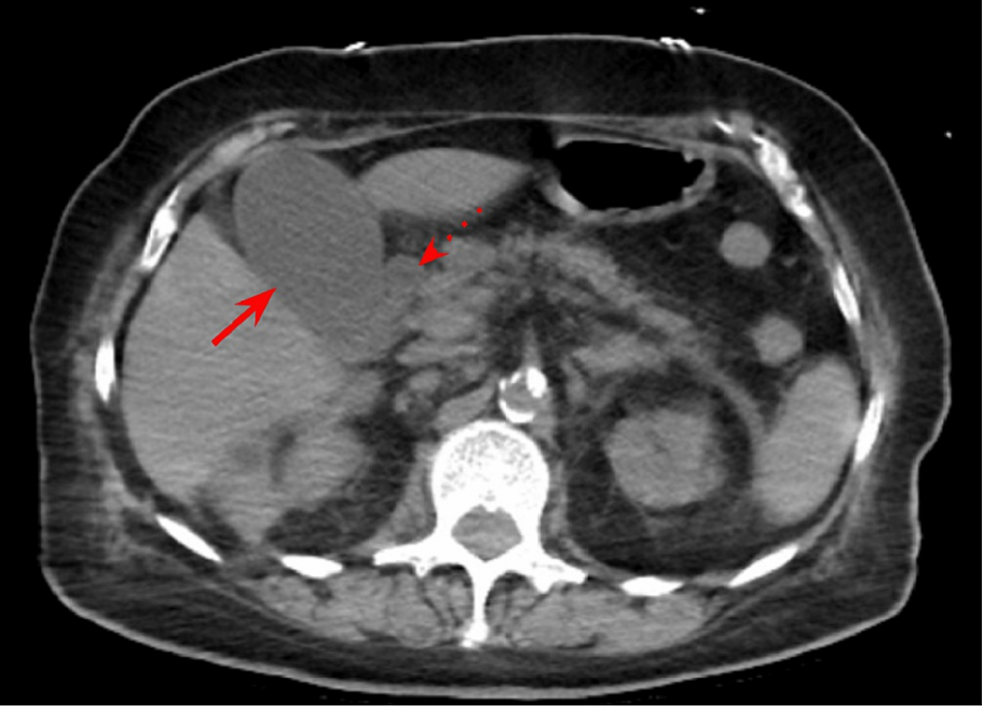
Initial CT abdomen and pelvis in the axial view CT abdomen and pelvis showed gallbladder distention (solid arrow) and increased prominence of the common bile duct (dashed arrow). No obstructing stone or mass.
